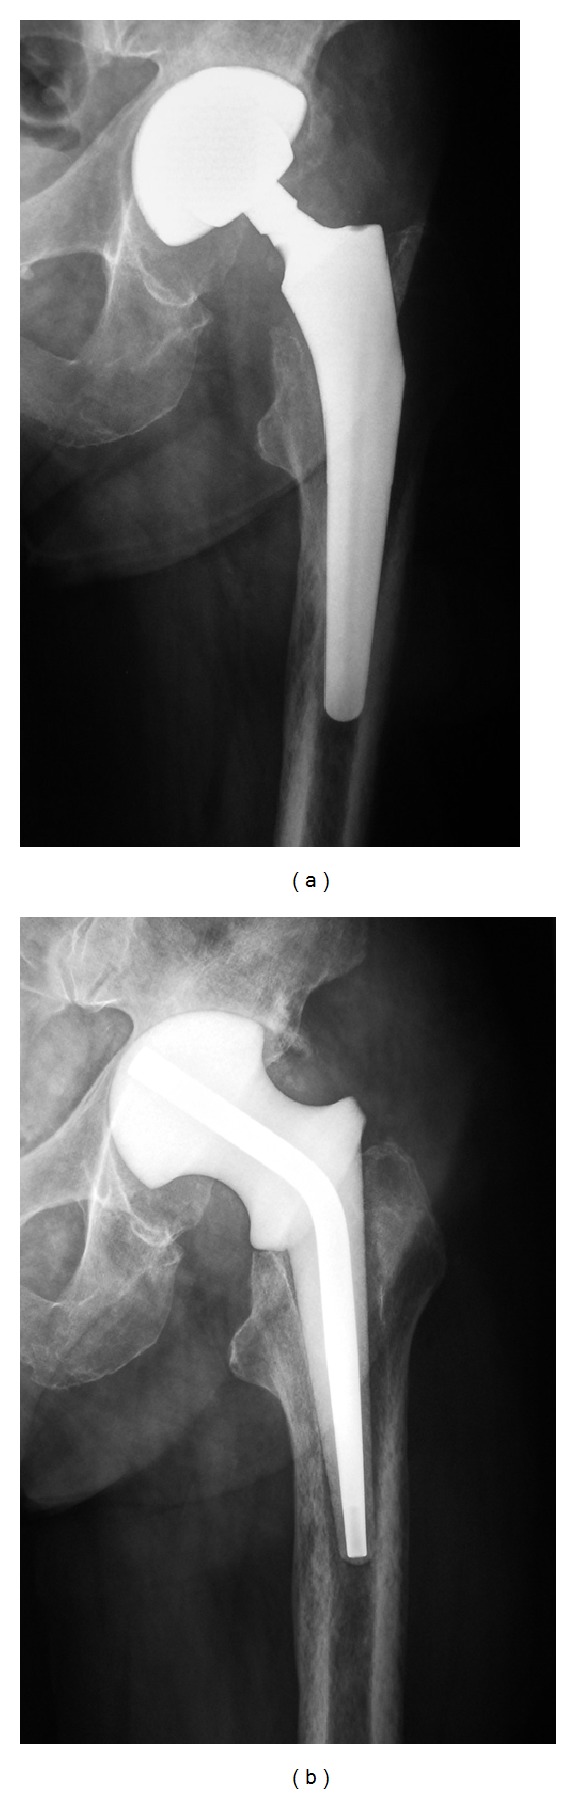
Preoperative X-ray of a total hip arthroplasty complicated by chronic infection (a). Radiograph obtained after removal of the infected prosthesis and implantation of an industrially manufactured spacer (b).
